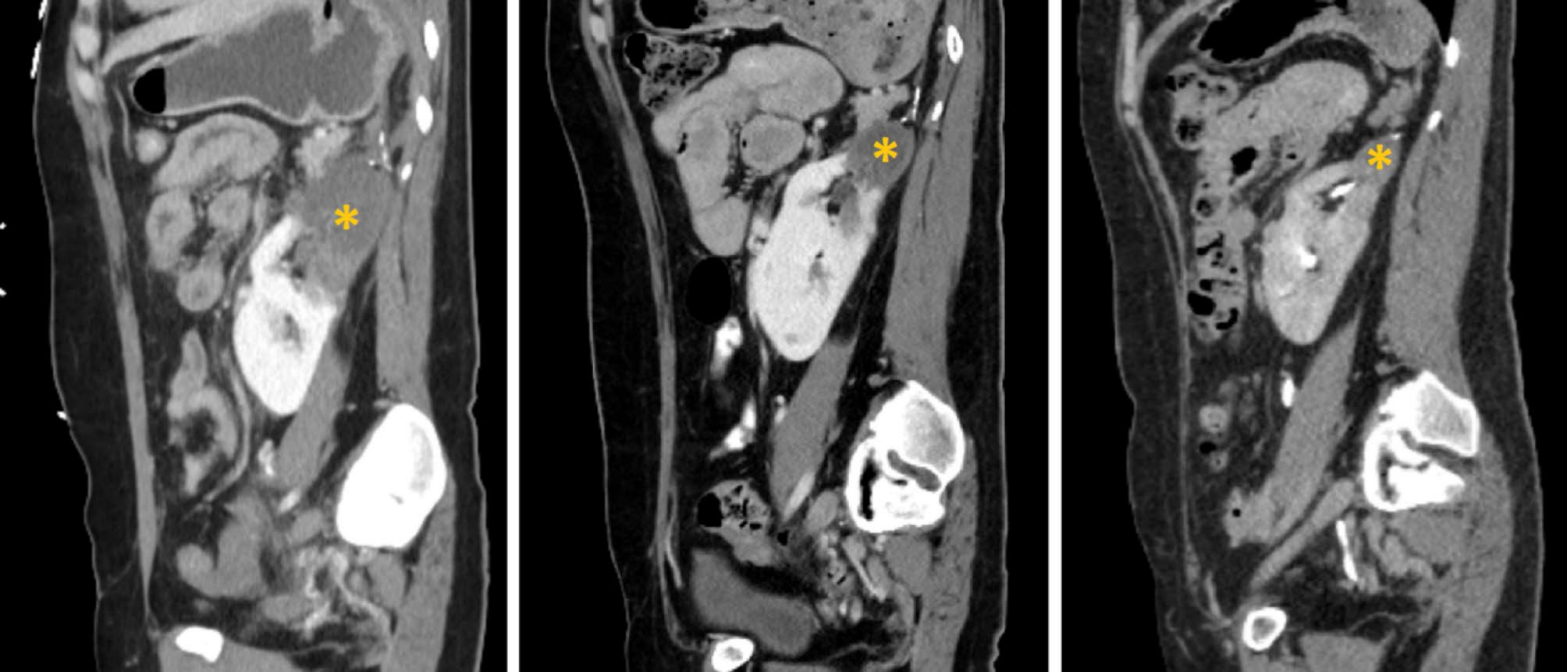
The postoperative contrasted CT chest/abdomen/pelvis in the sagittal plane demonstrating horseshoe kidney with improving hypoenhancing area in the left superior renal pole moiety (yellow asterisk), measuring 7.8 × 4.5 cm, 4.2 × 3.2 cm, and 2.1 × 1.7 cm at postoperative weeks of 1, 12 and 24, respectively
